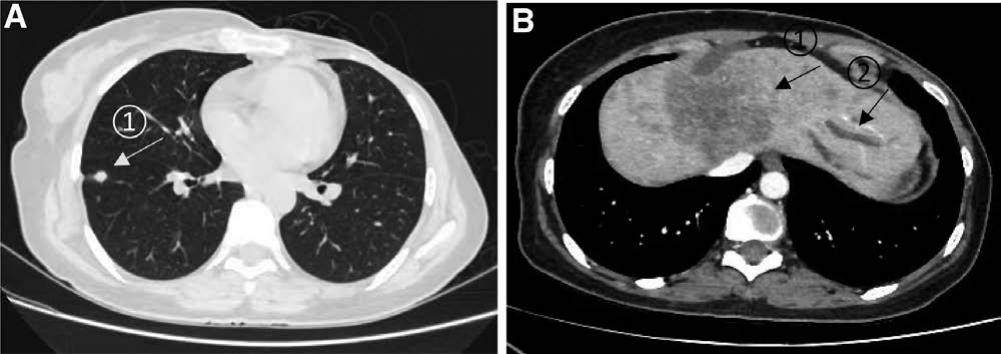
Computed tomography (CT) scan of (A) a small nodule in the lower lobe of the right lung, about 8 mm in diameter (arrow ①), considered to be a metastatic tumor, (B) multiple low-density lesions in the left lobe of the liver with a maximum diameter of approximately 73 mm (arrow ①), considered to be a metastatic tumor; obvious dilatation of the intrahepatic bile ducts was present in the left lobe of the liver(arrow ②).
